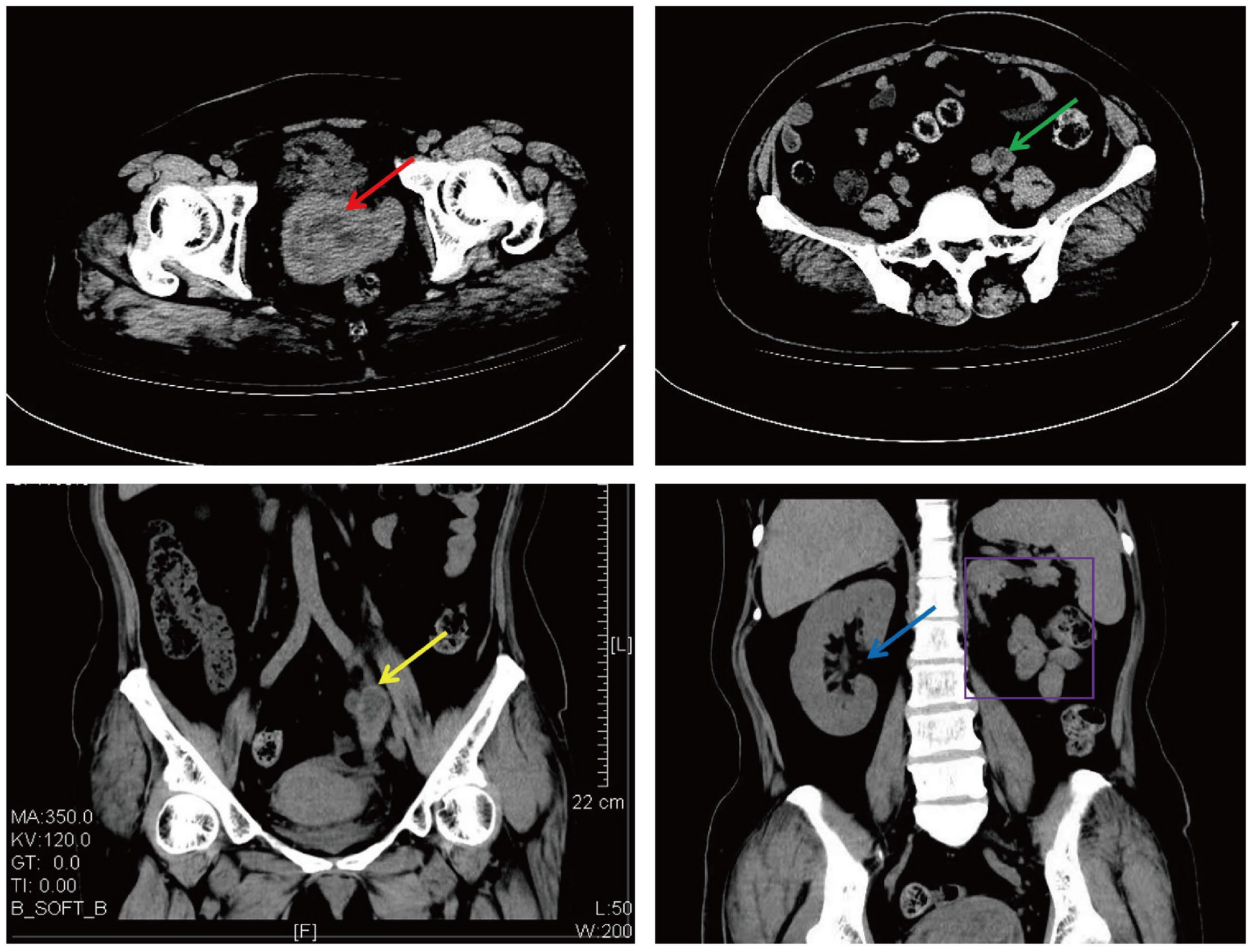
Cervical and left retroperitoneal mass shown on CT (The red arrow: a cervical mass; the green arrow: a horizontal left retroperitoneal mass; the yellow arrow: a coronal left retroperitoneal mass; the blue arrow: a coronal right kidney; and the purple border: a coronal left kidney absence).
